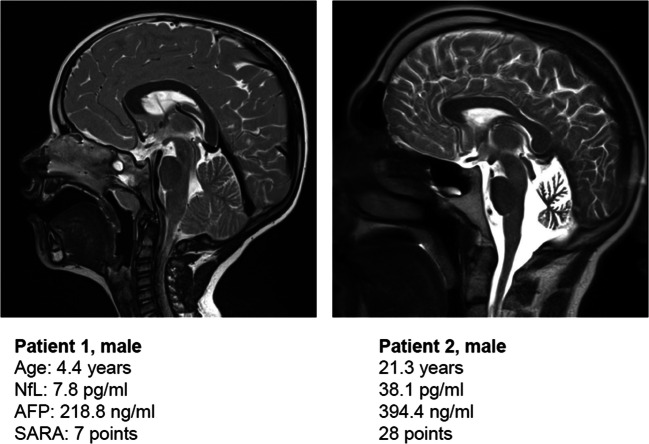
MRI scans (T2) of two A-T patients. Patient 1: a 4.4-year-old boy with mild neurological deficit, SARA of 7 points, and normal NfL. Patient 2: a 21.3-year-old man with severe neurological deficit, SARA of 28 points, and high levels of NfL
